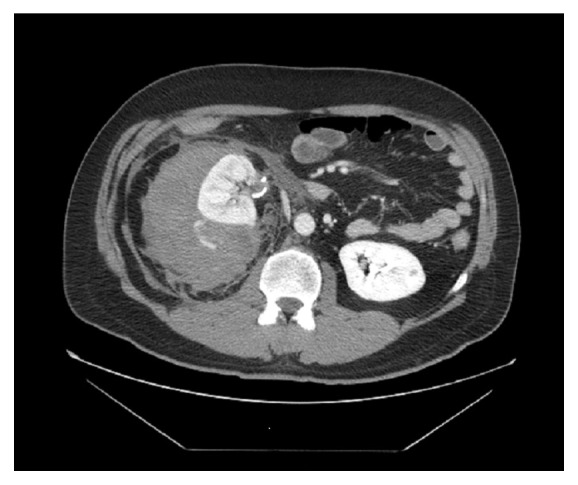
Active contrast extravasation from the right kidney on computed tomography.
